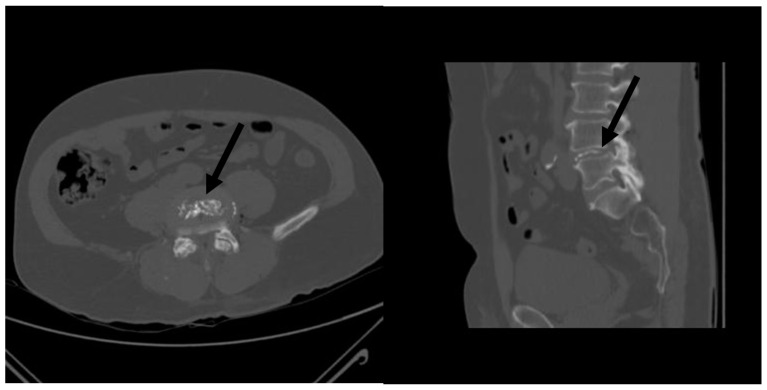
Axial and sagittal CT reconstructions performed immediately post RGE injection aiming to evaluate distribution of the product (black arrows) and rule out any potential extradiscal leakages.
